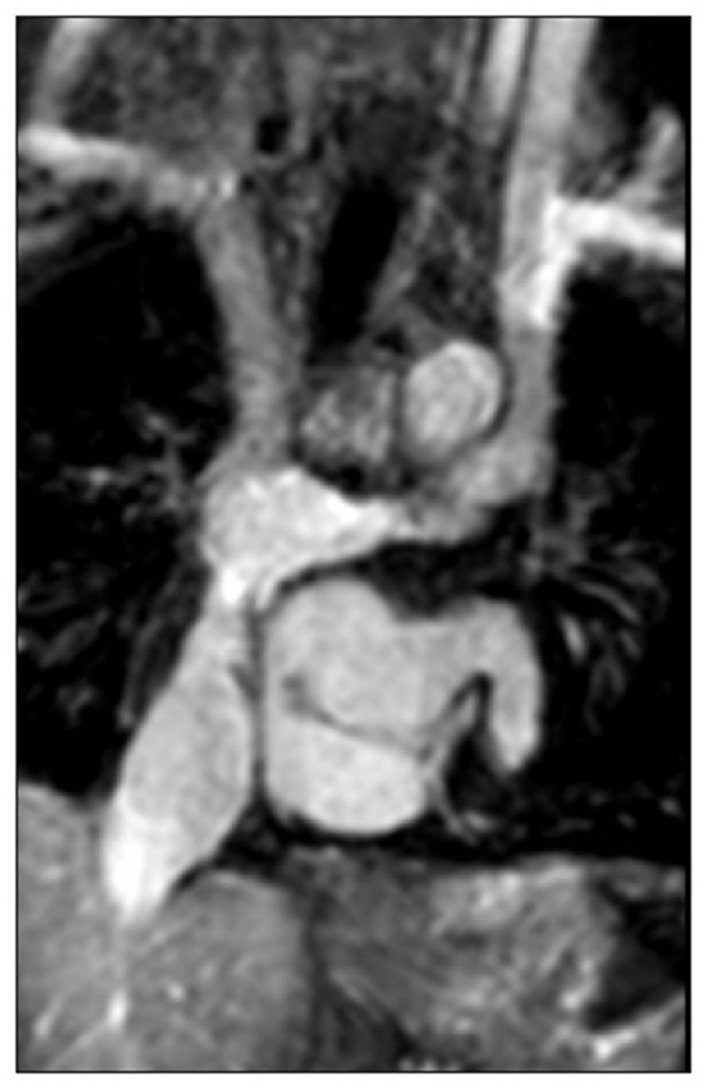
Narrowing of the Fontan circuit seen on MRI.
